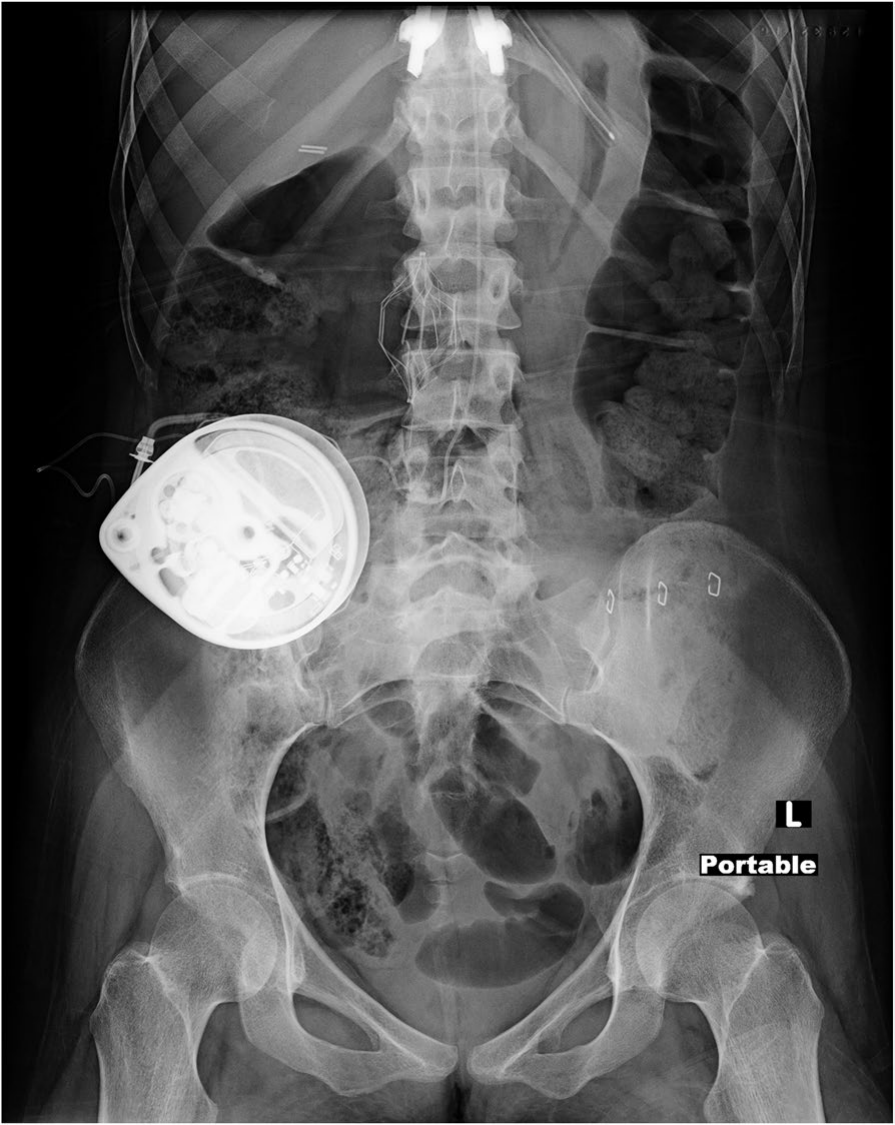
Plain abdominal x-ray showing baclofen pump with tubing present intrathecally
